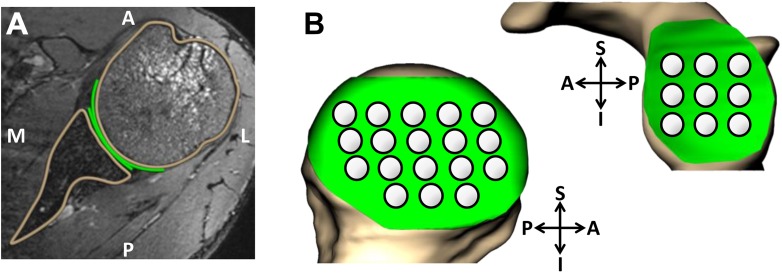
(A) Axial magnetic resonance image of a left shoulder. (B) Humeral head and glenoid articular cartilage grid systems for measuring regional changes in thickness. A, anterior; I, inferior; L, lateral; M, medial; P, posterior; S, superior.
